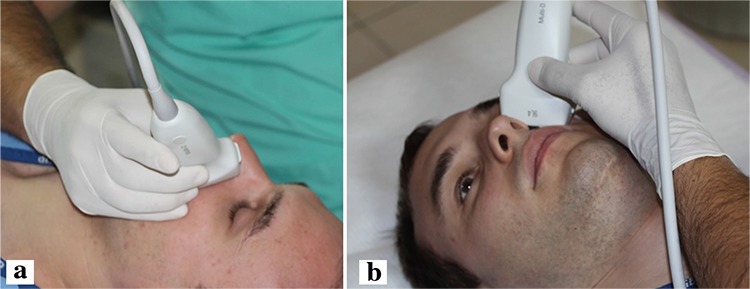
Patient and ultrasound probe positioning. The ultrasound elastography is performed from the lateral nasal wall.
